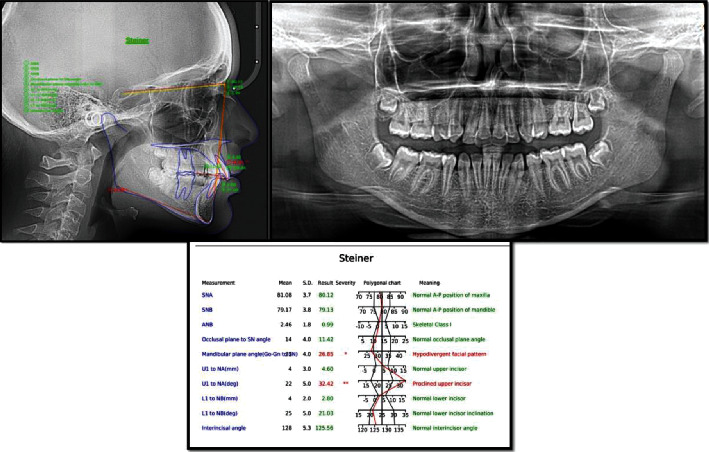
Pretreatment cephalogram and panoramic radiograph with analysis of the lateral cephalogram using the Steiner analysis.
